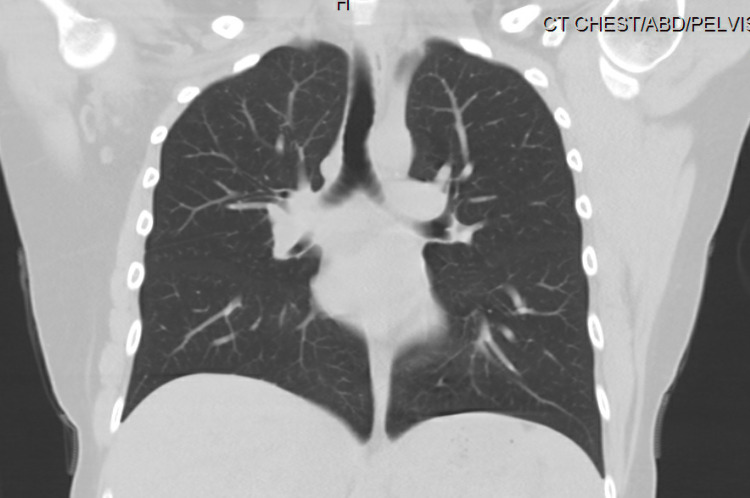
CT scan of the chest shows no evidence of mediastinal lymphadenopathy
